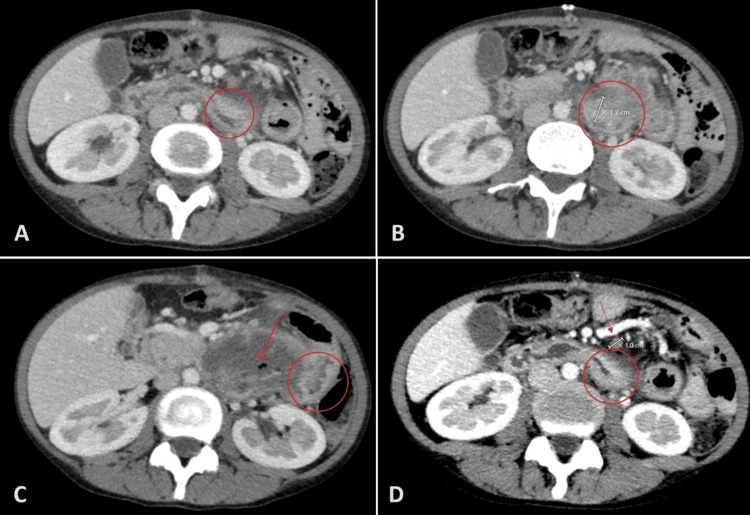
Abdominal CT scan with IV contrast showing irregular circumferential wall thickening of the proximal jejunal small bowel loops (2A, 1B), with a short segment appearing slightly dilated and fluid-filled (2C). Associated prominent regional lymph nodes are also noted measuring up to 1 cm (2D).
